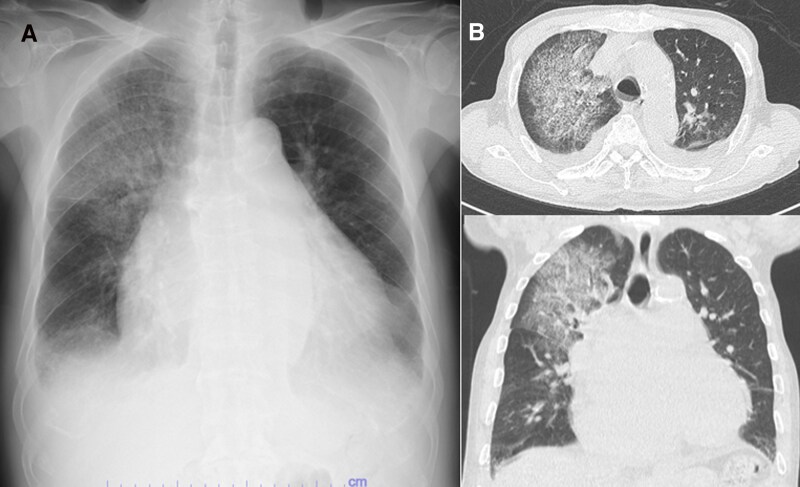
Chest radiograph and computed tomography findings on admission. (*A*) Chest radiography revealed marked cardiomegaly and extensive ground-glass opacity in the right upper lobe with partial consolidation. (*B*) Chest computed tomography revealed marked cardiomegaly with bilateral pleural effusions, extensive right upper lobe ground-glass opacity with partial consolidation, interlobular septal thickening, and relative subpleural sparing. The upper panel shows the axial view, and the lower panel shows the coronal view of the chest.
